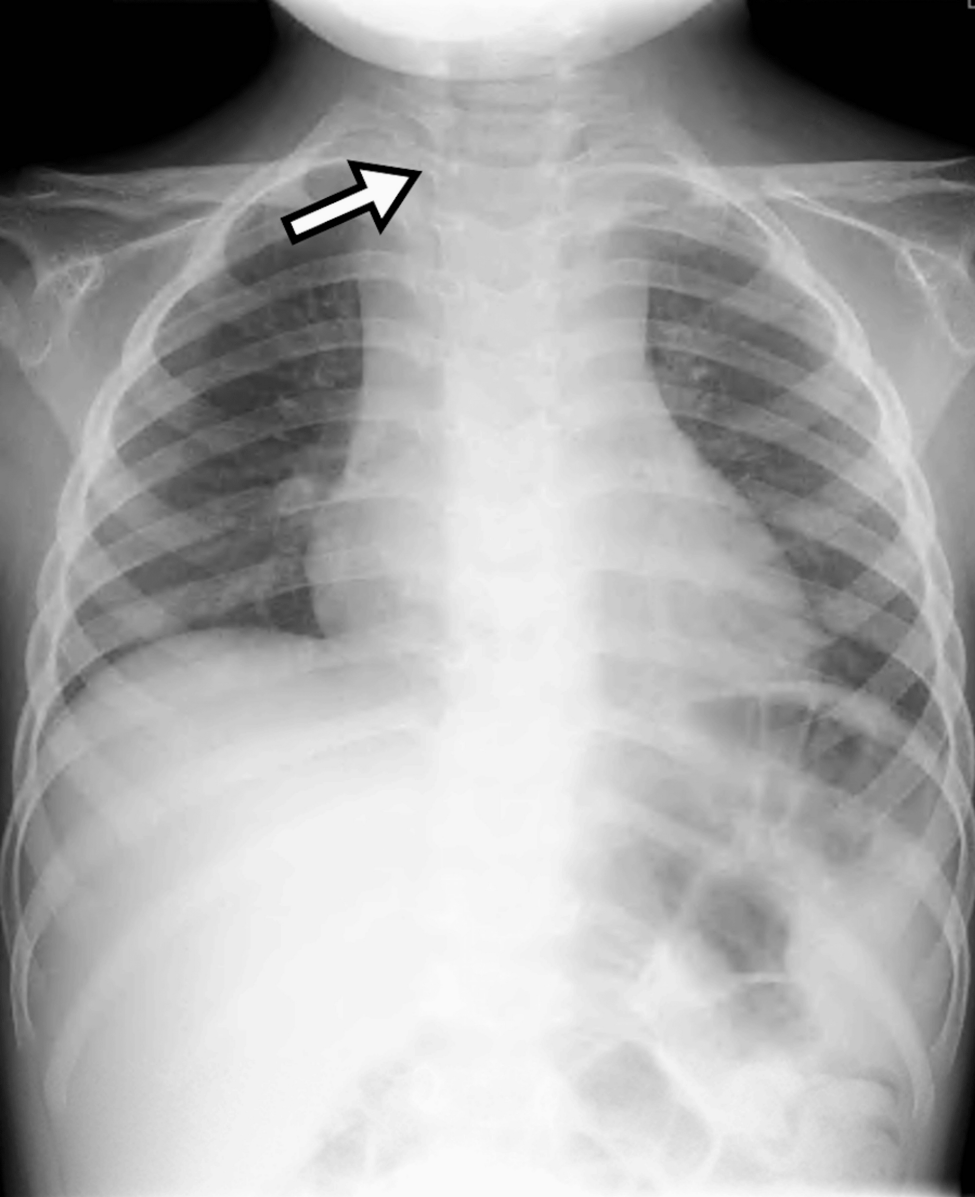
Chest radiography on the day of presentation. Chest radiography on the day of presentation showing rightward deviation of the trachea. The arrow indicates the displaced tracheal air column.
